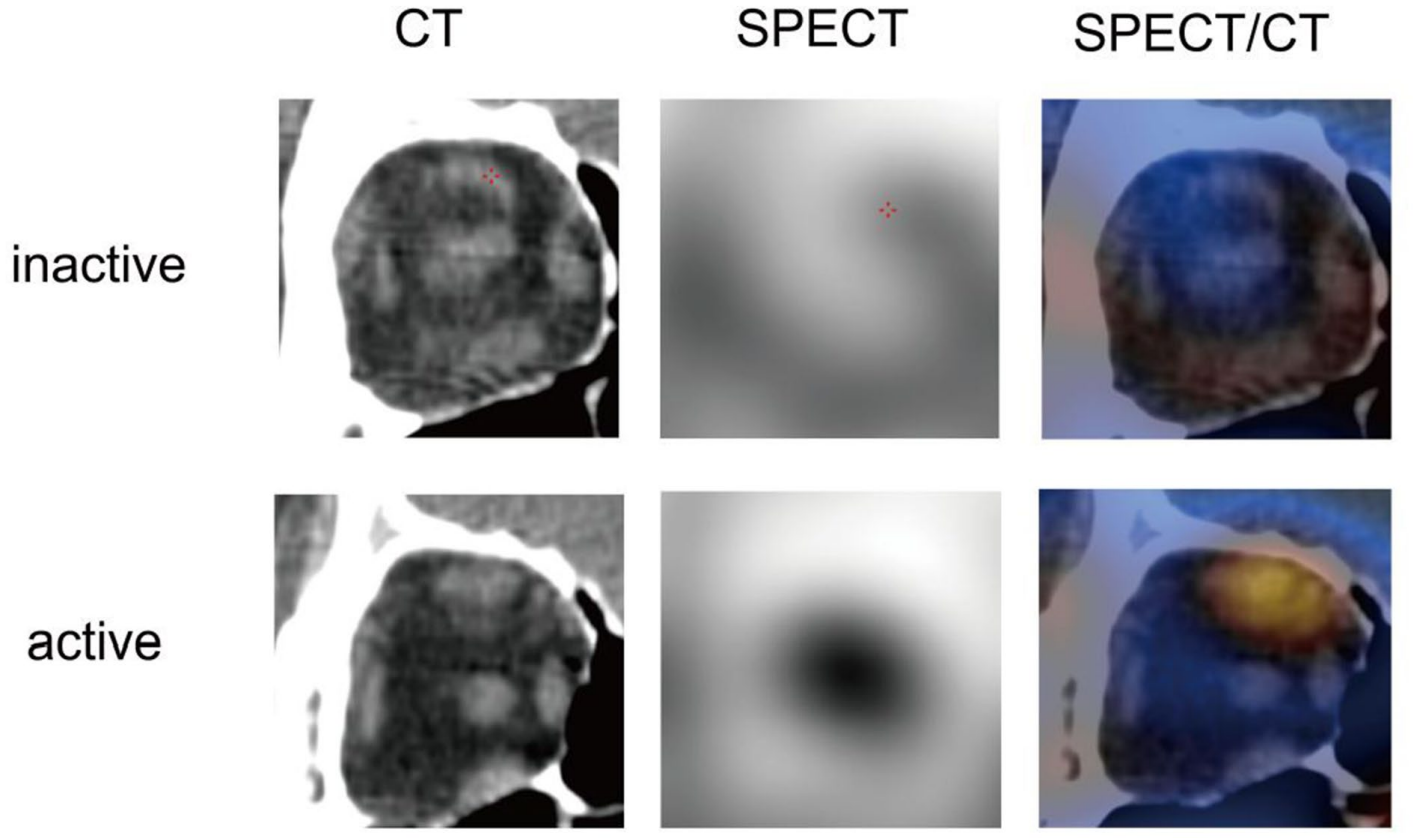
Representative unilateral radiographic manifestations of active and inactive TED defined by CAS.
